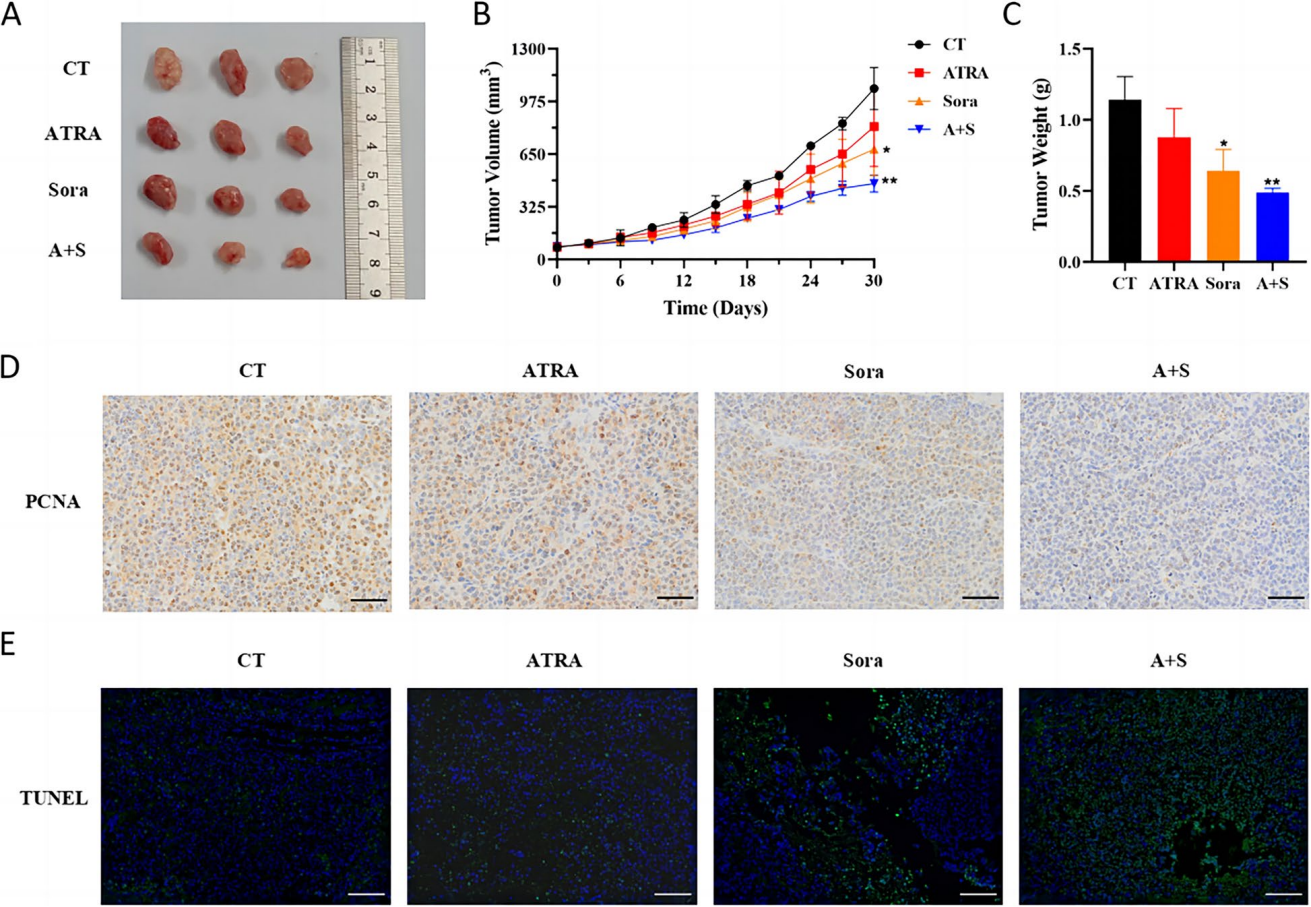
The combination of ATRA and Sorafenib most effectively inhibited cell line-derived xenografted tumor growth. HCC xenografted tumours were treated with ATRA and Sorafenib either alone or in combination as described in the materials and methods. A tumour image (A), and the tumour volumes (B) and weights (C) were presented. Tumour cell proliferation (D) was measured by the expression of PCNA in the xenografts as determined by IHC. Tumour cell apoptosis (E) was measured by TUNEL assay. CT, control; Sora, Sorafenib; A + S, ATRA combined with Sorafenib; p* < 0.05, *p* < 0.01, compared with CT, by t-test. Scale bar, 50 μm
